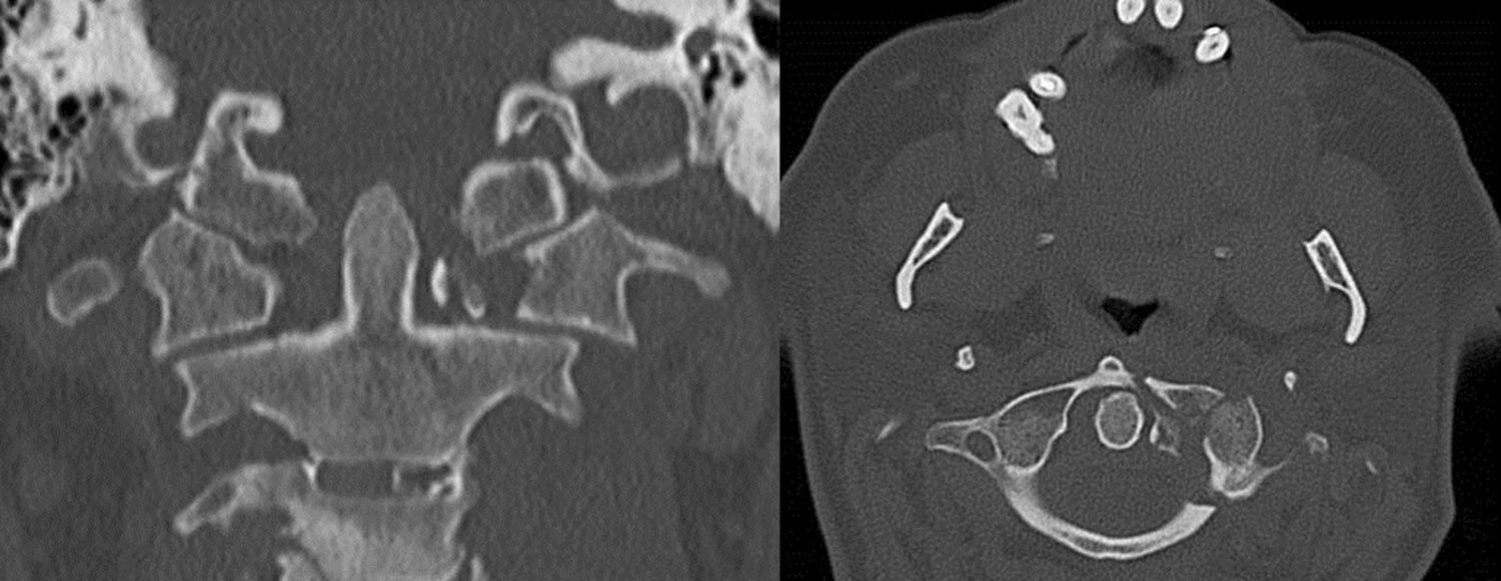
AP (*left*) and transversal (*right*) CT images of the upper C-spine of patient 1. Fracture of the left lateral mass of C1 (Jefferson type IV, Landells and Van Peteghem type III; Fig. 5) with dislocation of 7 mm
